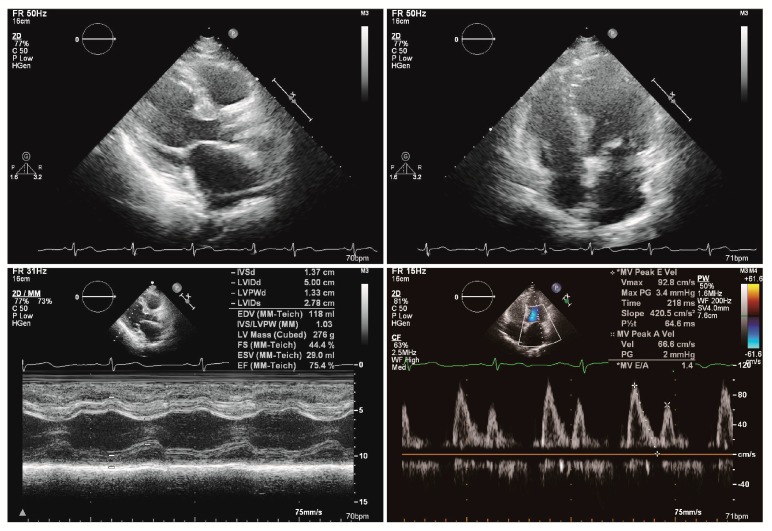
The cardiac ultrasound showed normal wall motion with a left ventricular ejection fraction of 75.4%. There was no significant valvular disorder.
